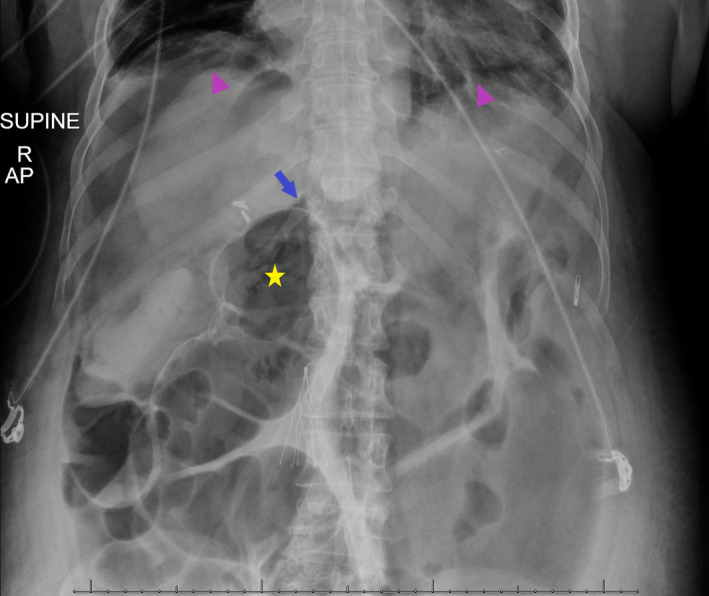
Abdominal radiograph in a supine position demonstrating classic signs of pneumoperitoneum – double dolphin sign (arrowhead), triangle sign (arrow), and double wall (Rigler) sign (star)
